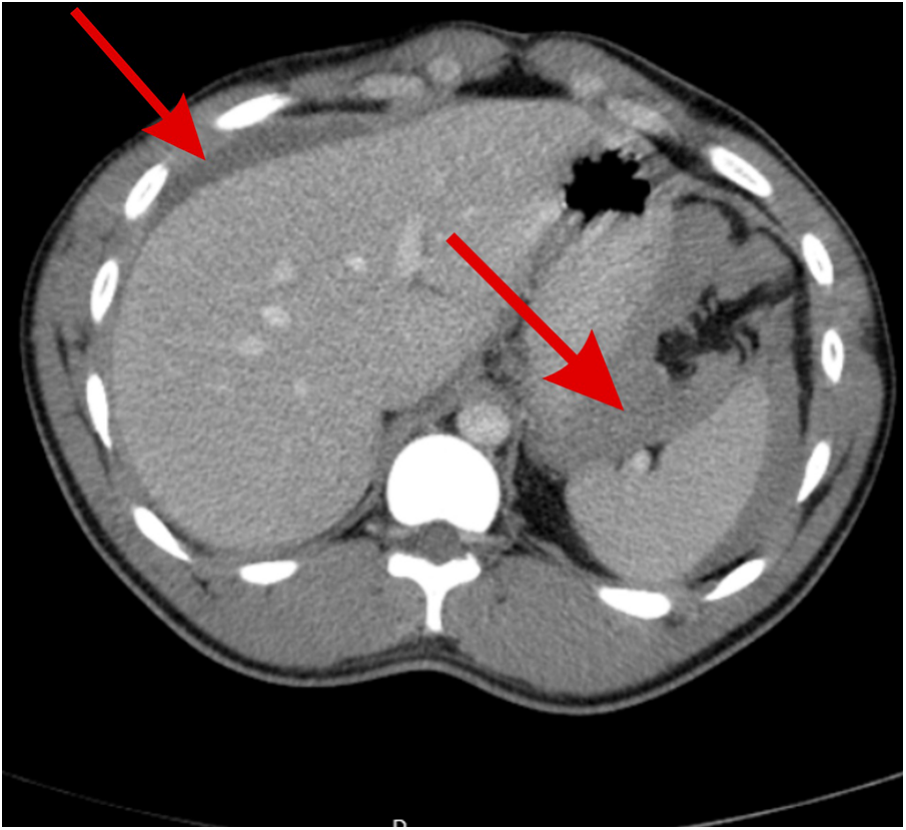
Abdominal CT scan showing free fluid of blood density around the spleen, between the spleen and stomach, and around the hepatic areas, indicating hemoperitoneum (red arrows).
